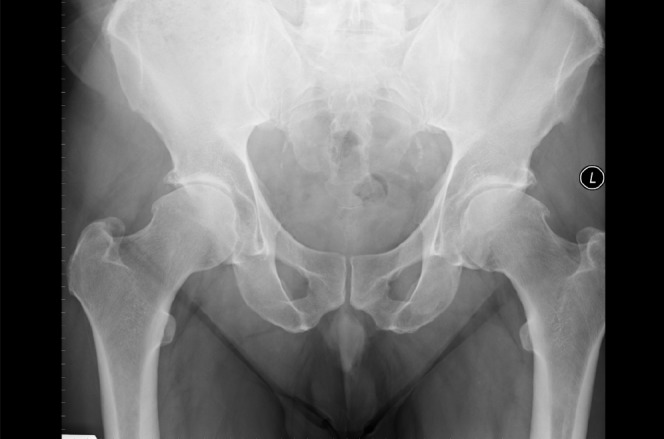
Anteroposterior x-ray of the pelvis with bilateral hip osteoarthritis
